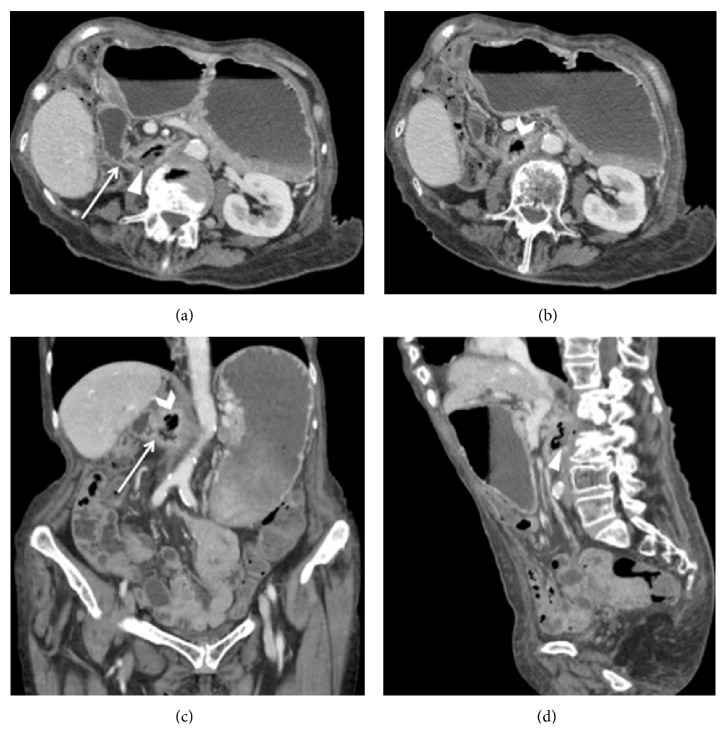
Follow-up contrast-enhanced MDCT study performed three months later. Axial ((a) and (b)) and coronal (c) CT images well show the presence of a fluid-filled fistula (white arrows (a) and (c)) between the duodenum and the IVC graft and an air bubble collection inside the prosthesis (open arrows (b) and (c)). Axial (a) and sagittal (d) CT images better show the path of enteric fluid into the IVC graft. Dilatation of the stomach with an air-fluid level and thickening of the duodenum are also evident.
